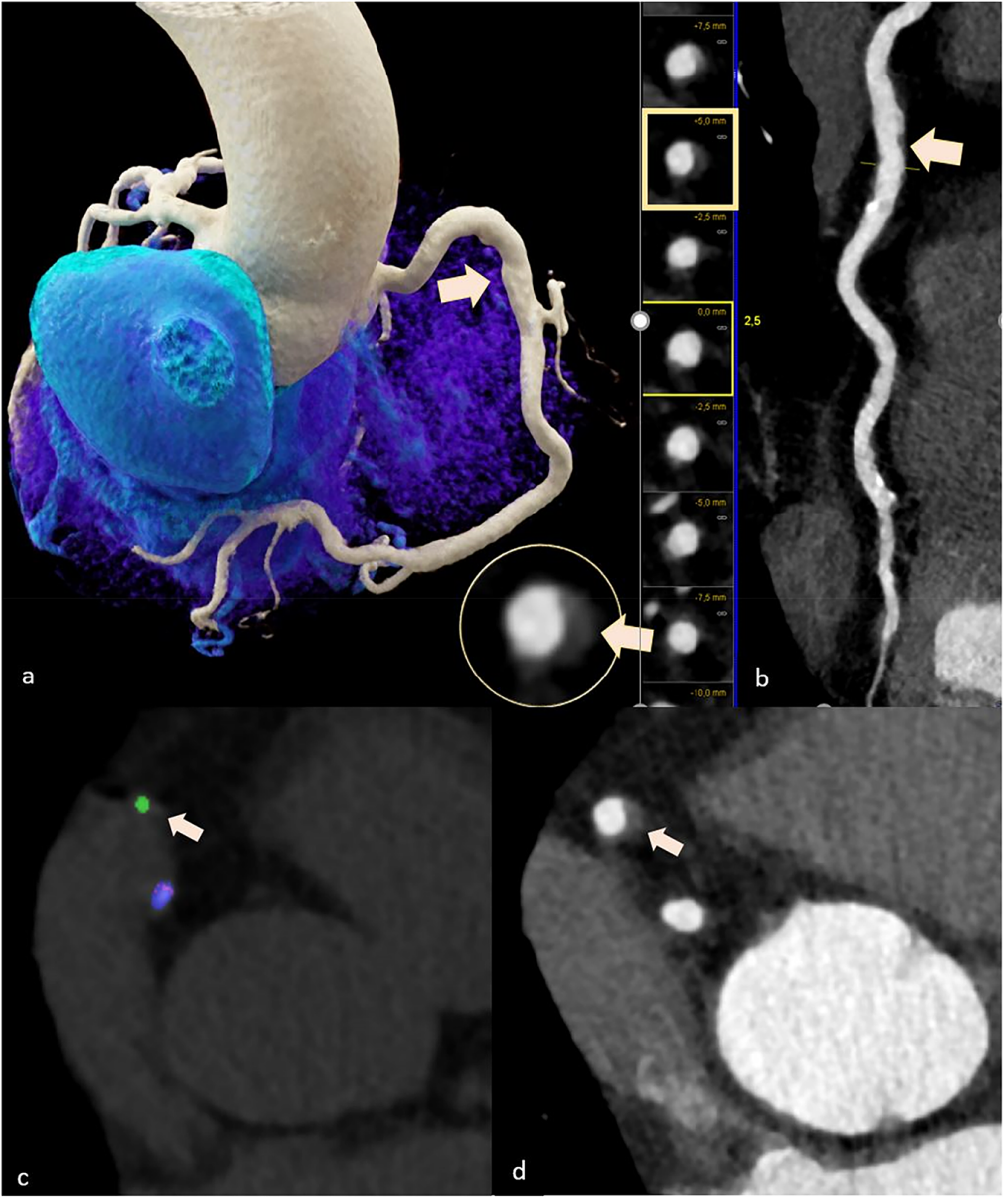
A 63-year-old male with gout and a high-risk low attenuation plaque (28–50 HU and positive remodeling) (arrows in a, b, and d) in the proximal RCA, which correlated with an MSU-positive lesion (arrow in c). Three-dimensional volume rendering technique (a) and curved multiplanar reconstruction (b). DECT scan (c). d Coaxial CTA image alignment of the proximal RCA (d). Green = MSU-positive lesion. In this patient, the CAC score was 565 Agatston units. CAC, Coronary artery calcium; DECT, Dual-energy computed tomography; MSU, Monosodium urate; RCA, Right coronary artery
